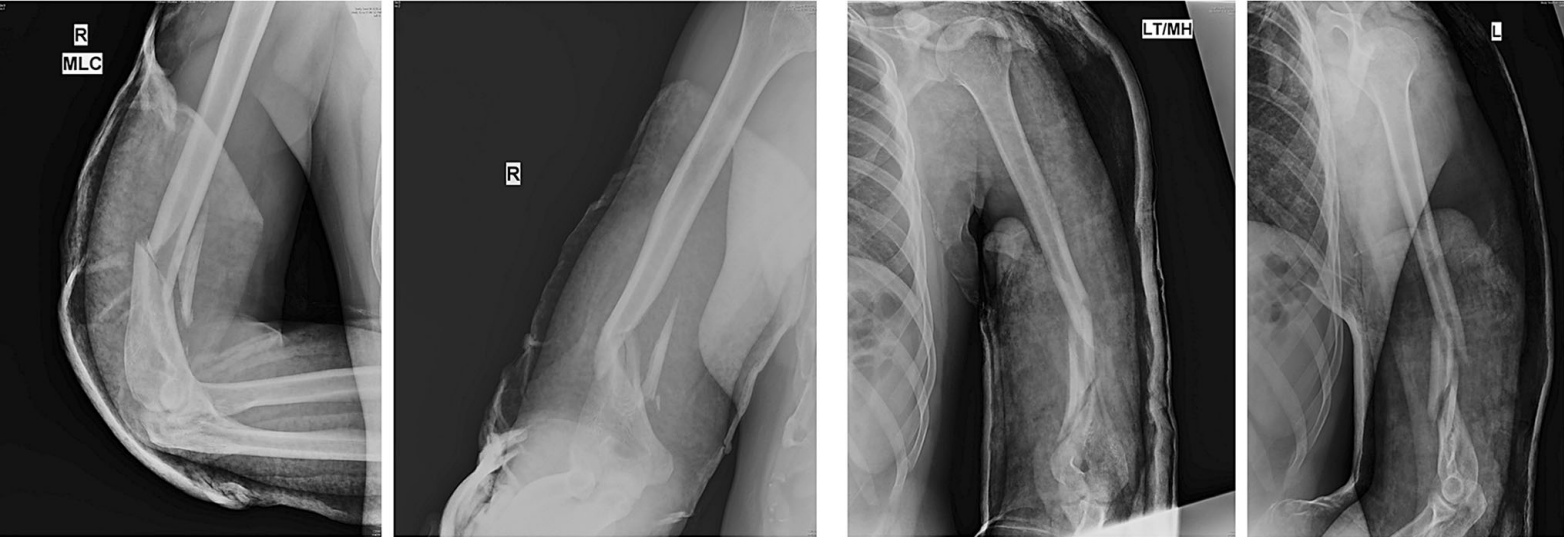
Showing AP and lateral views of X-rays with low distal humeral “extra articular” fracture
